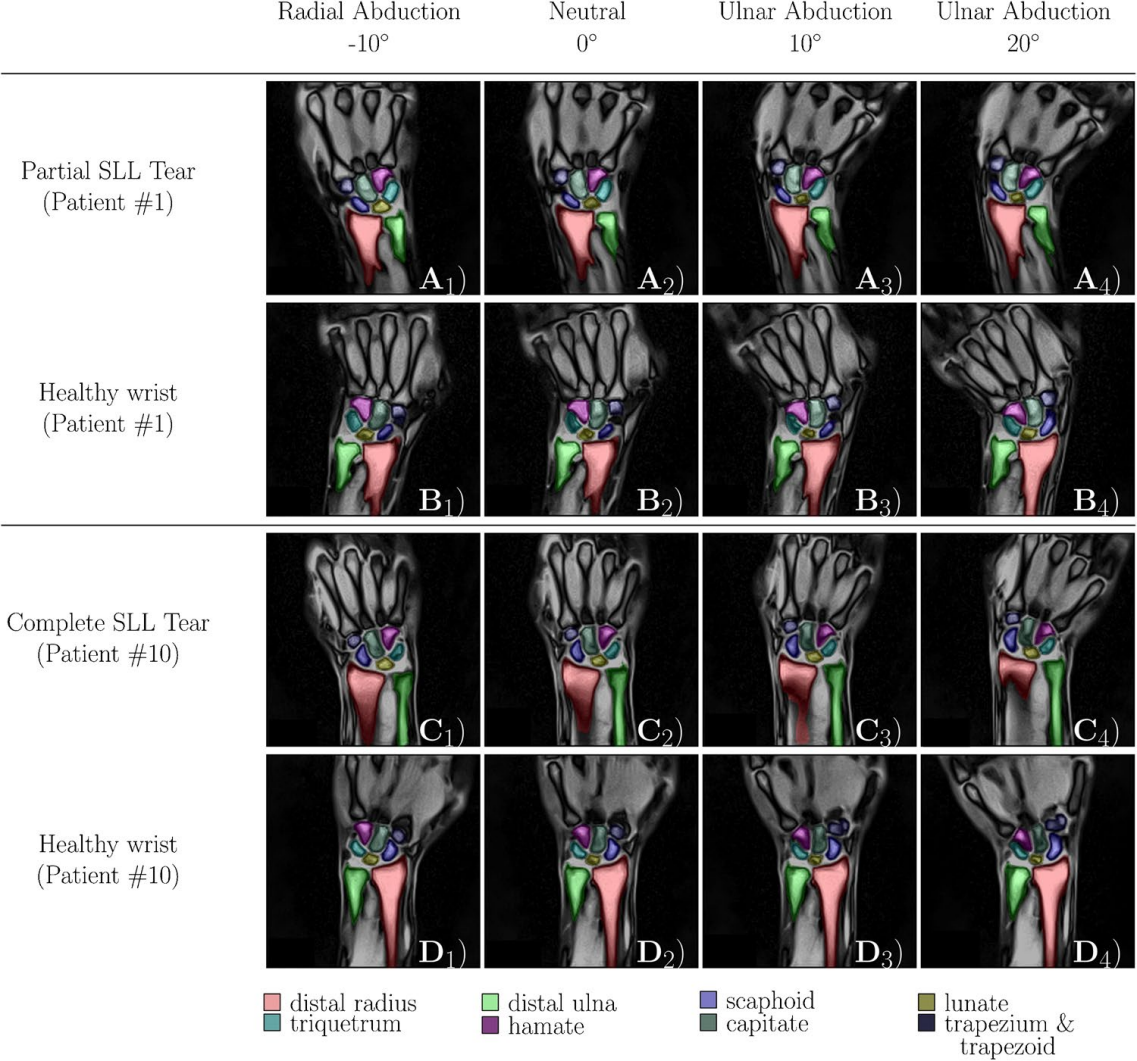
Visualization of injured and healthy wrists in different wrist positions as a function of scapholunate ligament condition. Displayed are selected real-time MR images in two exemplary patients. Patient #1 had a tear of the dorsal scapholunate ligament (SLL). His injured (A_1_-A_4_) and contralateral healthy wrists (B_1_-B_4_) are shown. Patient #10 had a complete SLL tear (C_1_-C_4_, D_1_-D_4_). The forearm and carpal bones were segmented automatically and color-coded as detailed below
